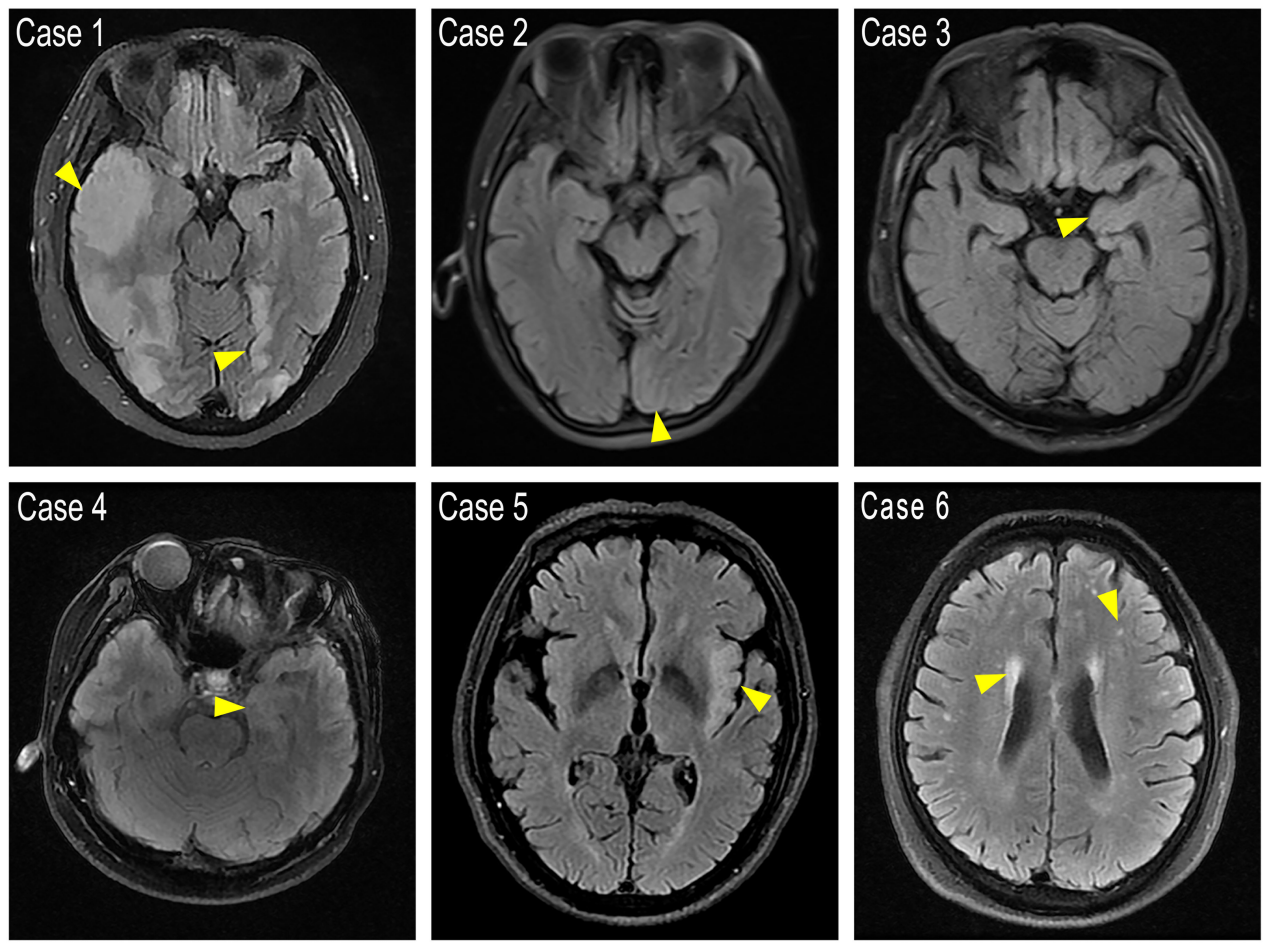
FLAIR images showing cortex abnormal signals. Case 1: Abnormal high signal was found in the bilateral frontotemporal occipital lobe, right parietal lobe, and left insular lobe with main involvement of the cerebral cortex. The boundary between gray and white matter is unclear. Case 2: A hyper-intensity in the left occipital cortex with swelling of the gyrus. Case 3 and Case 4: Left hippocampus showed slight hyper-intensity. Case 5: Bilateral frontal lobes, left temporal insular lobes present with abnormal signals. Case 6: Representative demyelination case with bi-ventricular zone, parietal temporal lobe with matter dots-like hyper signals. Yellow arrows point to the abnormal high intensity areas.
